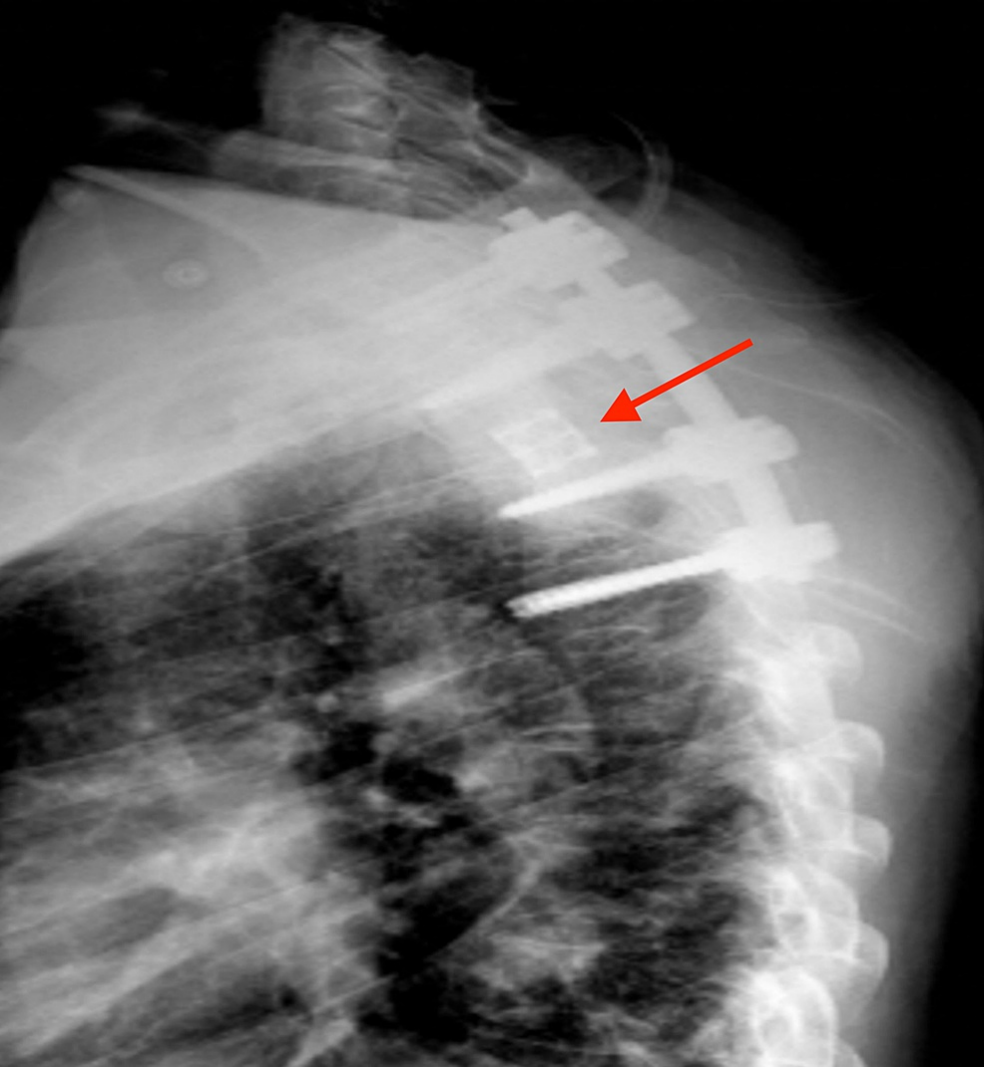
A postoperative lateral radiograph showed bilateral pedicle screw instrumentation from C7 to T4, T2 corpectomy, and placement of interbody mesh cage.
